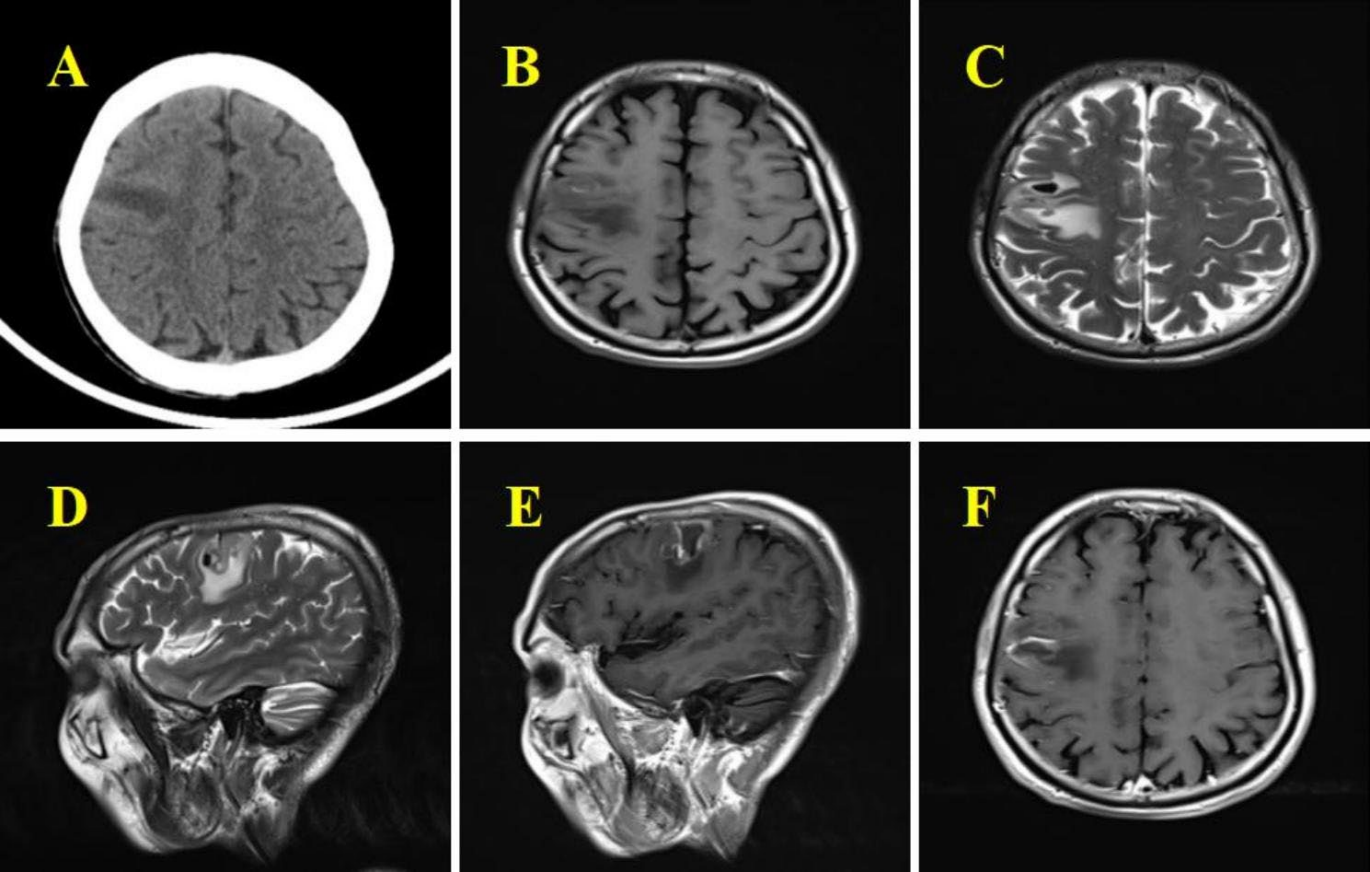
Imaging data of the patient. A. Axial CT scan of the brain showed low density lesions in the right frontal lobe. B-D. Axial T1-weighted images, T2-weighted images and sagittal T2-weighted images of head MRI were shown respectively: equal/slightly longer T1 long/short T2 abnormal signals were observed in the right frontal lobe (the anterior central gyrus was dominant), and flacy-like edema signals were observed around the lesion. E-F. Are the axial and sagittal enhanced images of brain MRI respectively: the edge of the lesion was significantly enhanced in the enhanced scan
